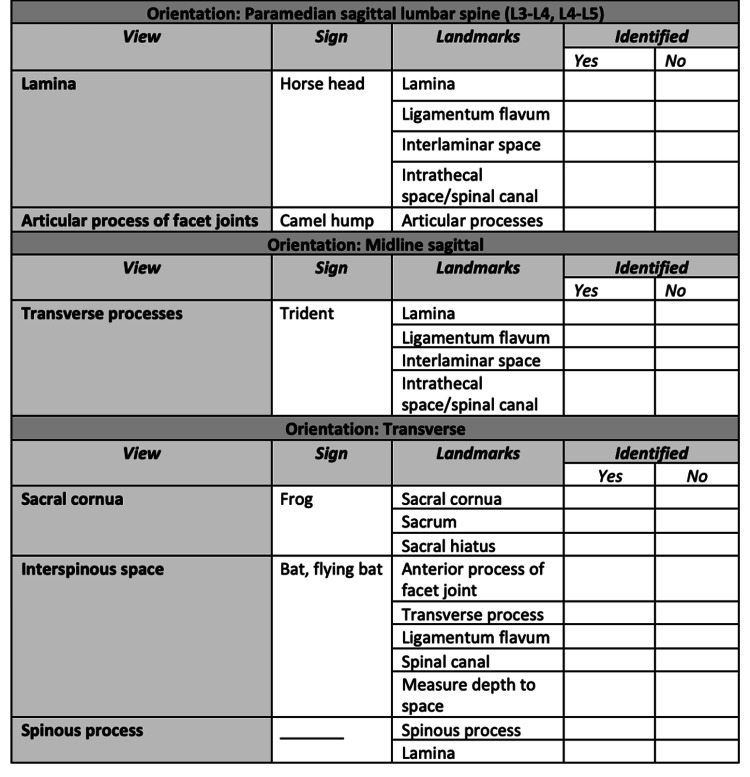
Qualitative assessment tool used by evaluators to track participants’ ability to identify all specified landmarks associated with each neuraxial ultrasound view.
